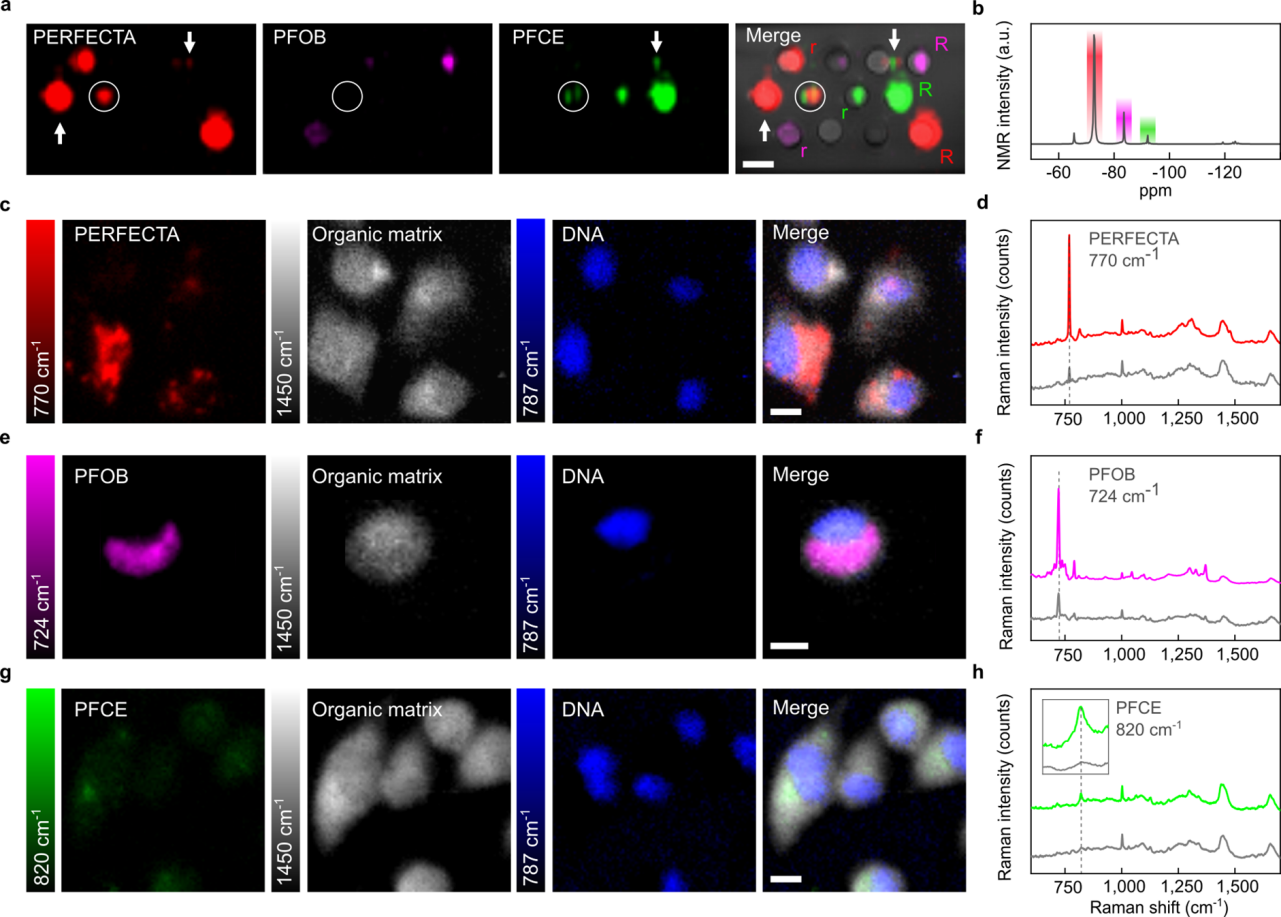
Raman and ^19^F-MRI multicolor imaging of labeled cells.
